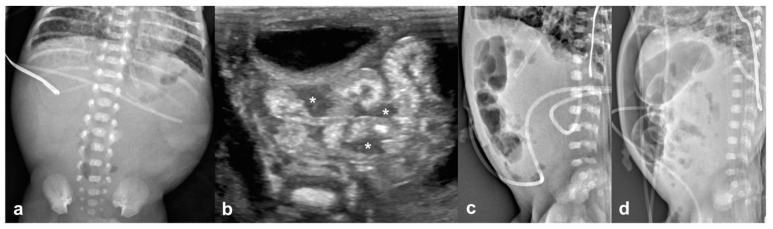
21-day-old boy (born at gestational age of 23 weeks and 2 days) with perforated necrotizing enterocolitis and radiographic pneumoperitoneum; this infant died 38 days after laparotomy. (a) An abdominal radiograph obtained 1 day before US shows a relatively gasless abdomen without pneumoperitoneum. (b) Preoperative US images show increased intestinal wall echogenicity and wall thickening, and complex ascites (asterisks). (c) Preoperative abdominal radiograph obtained 4 h after US showed no free air. (d) Pneumoperitoneum appears on abdominal radiographs obtained 4 days after US.
